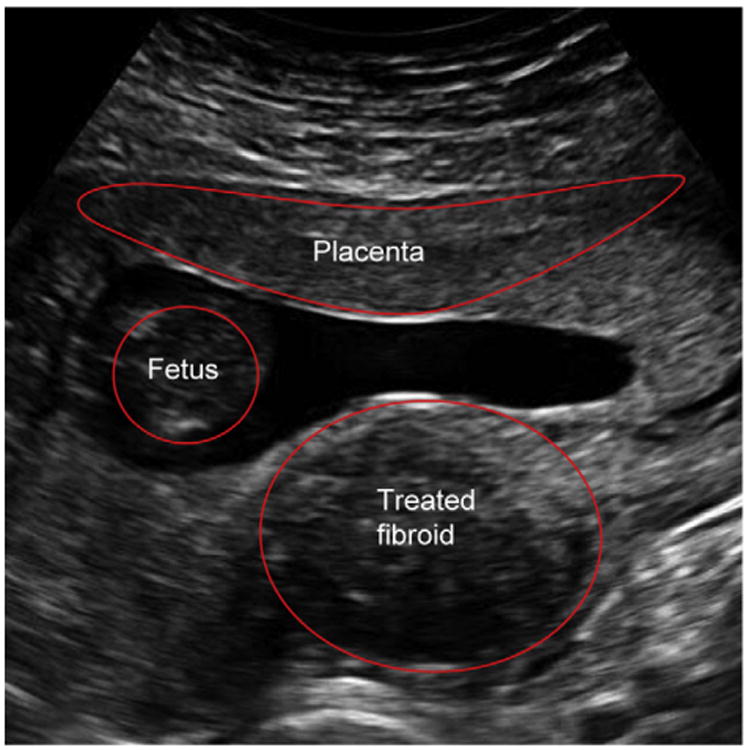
Transabdominal ultrasound image at 12 weeks' gestation showing the implantation of the placenta on the anterior wall. The treated dominant fibroid is located in the lateral myometrial wall and abuts the endometrial cavity.
